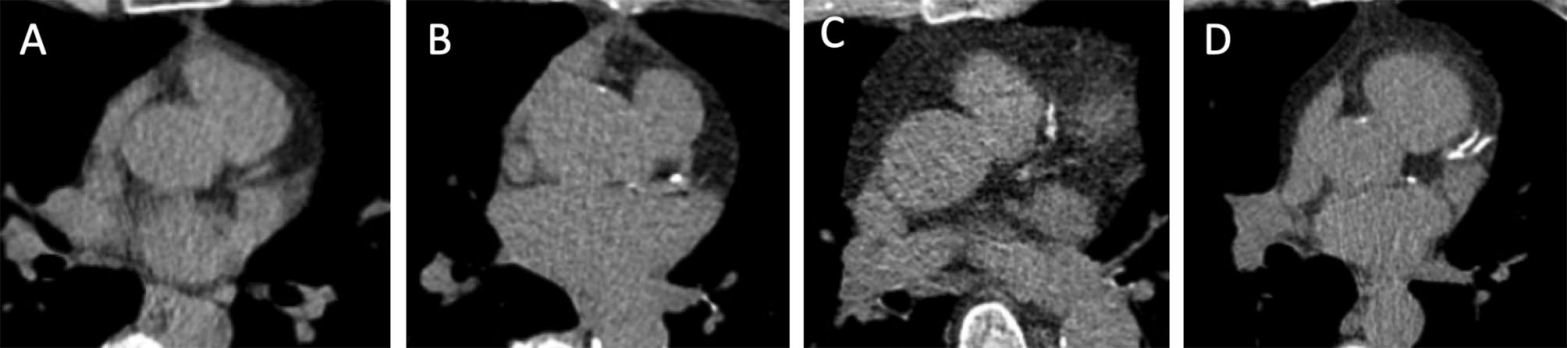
A–D. Collateral (smoking-related) findings in screening LDCT. Calcifications of the coronary arteries. Axial CT images at the level of the left main coronary artery showing different degrees of coronary artery calcification (CAC): absent = 0 (A), mild = 1 (B), moderate = 2 (C) and severe = 3 (D). According to the scale proposed by Chiles et al. [67], isolated flecks correspond to a mild degree (B), continuous calcification along the vessel correspond a severe degree (D)
